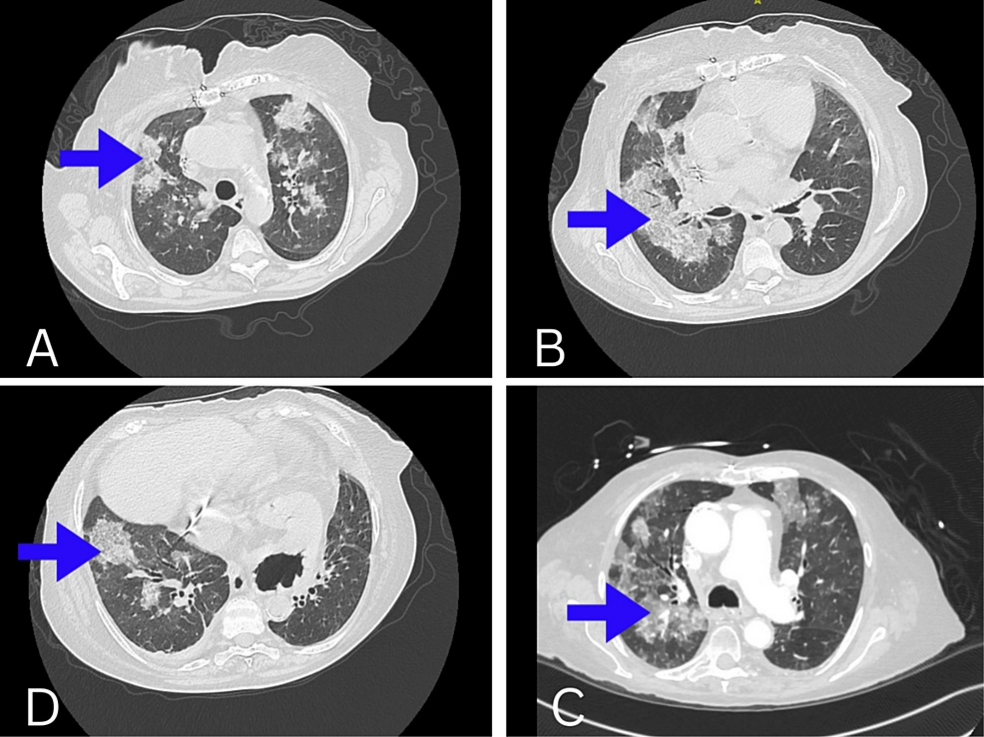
Preoperative computed tomography scan chest images showing ground-glass opacity in the upper lobe (A), middle lobe (B, C), and lower lobe (D).
